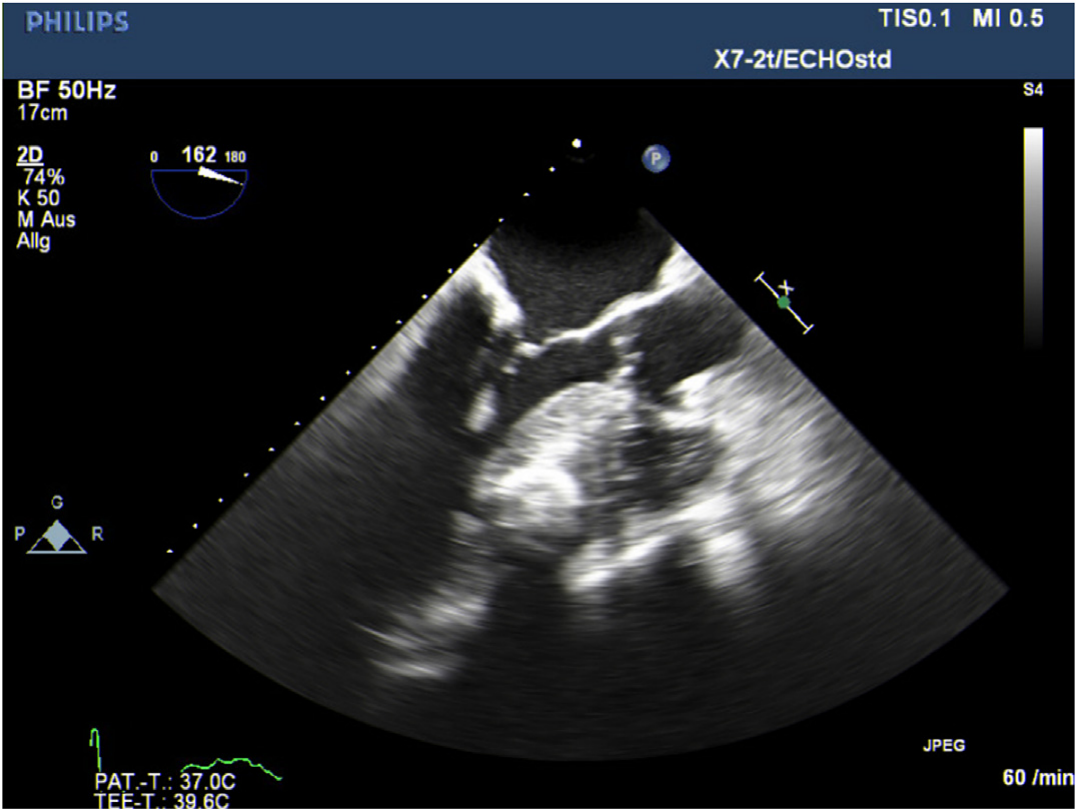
Echocardiographic Findings of Massive Myocardial Calcification Transesophageal echocardiography showing a significant septal left ventricular hypertrophy with calcified myocardium and a vegetation on the posterior mitral leaflet.
